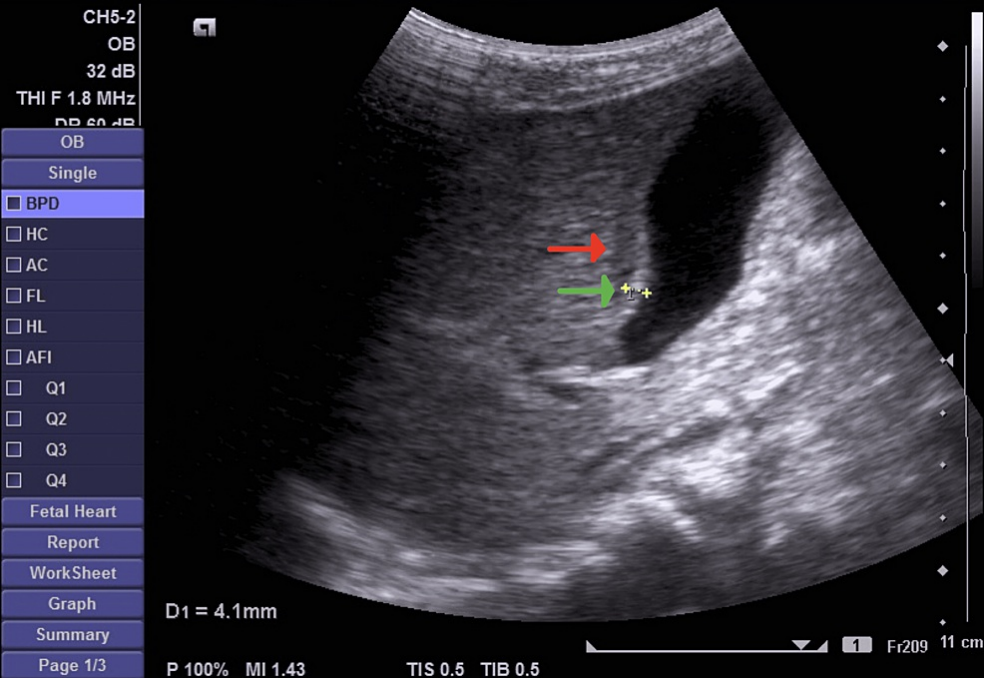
Abdominal ultrasound Red arrow: pericholecystic free fluid, green arrow: gallbladder wall thickness (4 mm)
